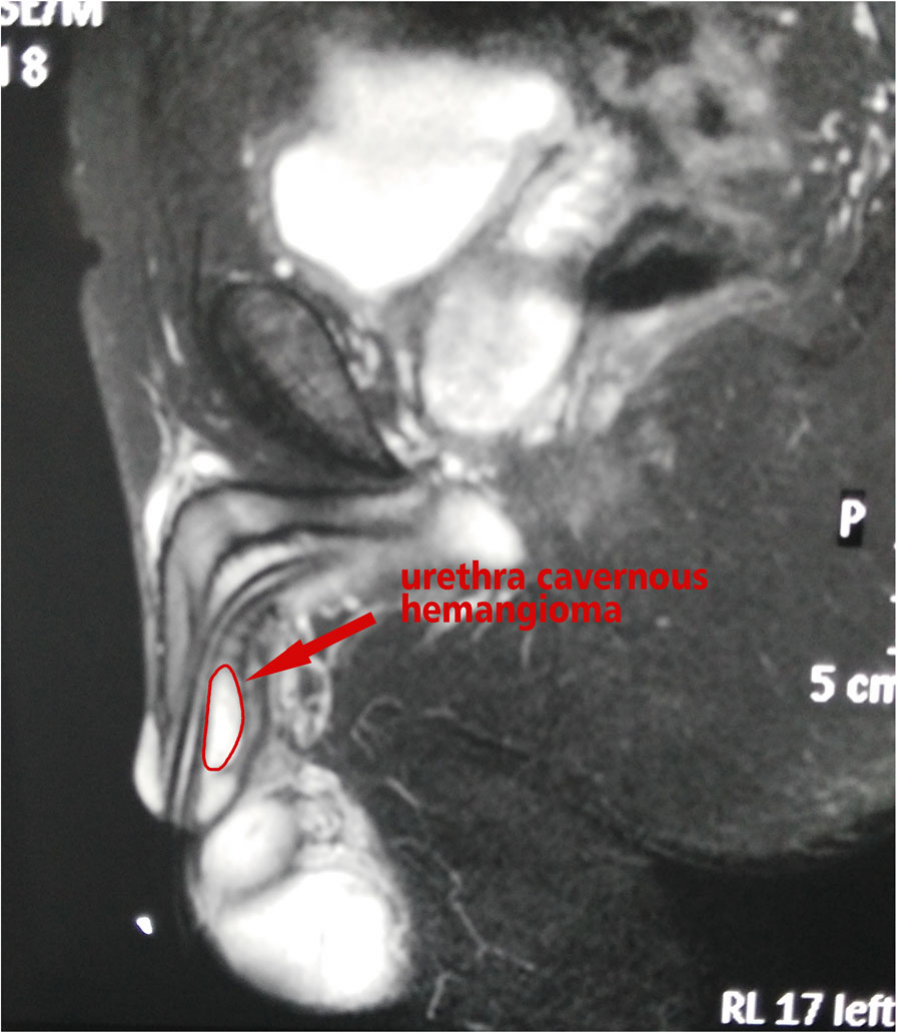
Preoperative penis sagittal MRI shows a high signal on the urethra cavernous body at the front of the penis. The range was about 1.1 × 2.4 cm
